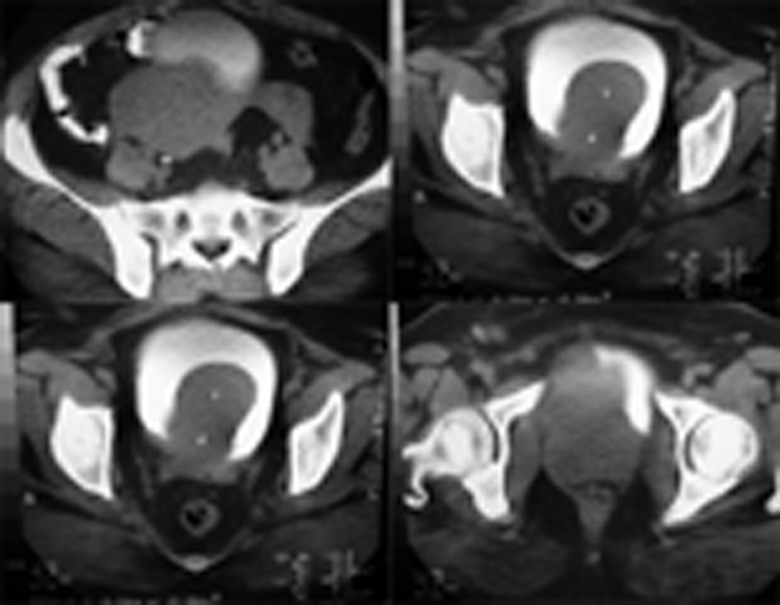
CT Scan Shows Multiple Solid Masses Between Bladder, Uterus and Vagina
